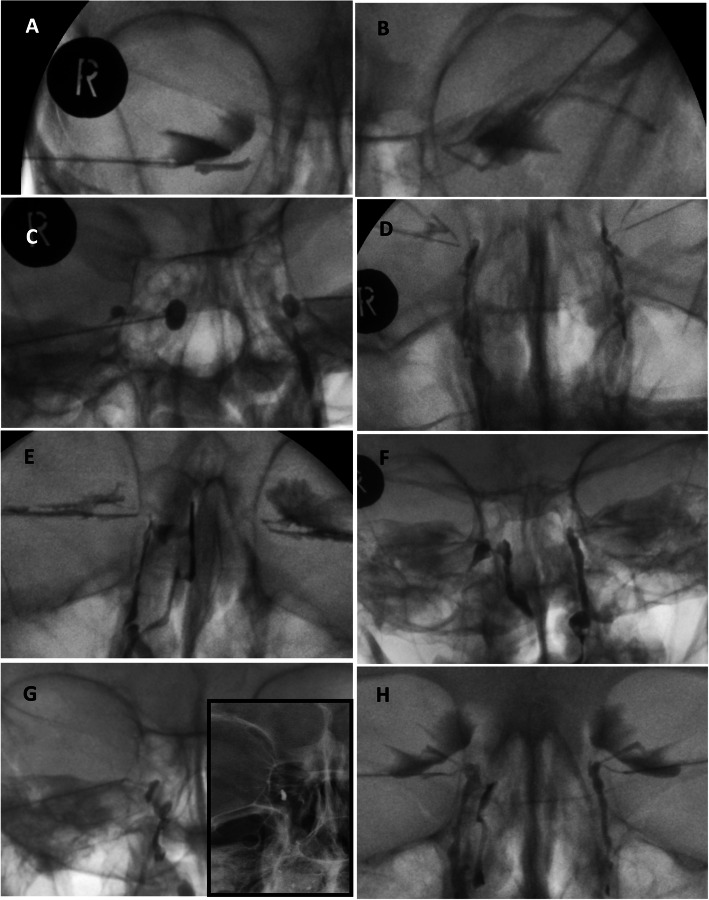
Images showing the different abnormalities seen on DCGs following DCR surgery. a right inferior canalicular block, b left common canalicular block, c right complete surgical anastamosis closure with closed sac remnant, d left complete surgical anastamosis closure with flow through the nasolacrimal duct, e right narrow anastomosis with narrow flow-stream into the nasal space, f right narrow and high anastamosis, g right narrow anastamosis with retained dye on erect x-ray, h right narrow anastamosis with dye also passing through the nasolacrimal duct
